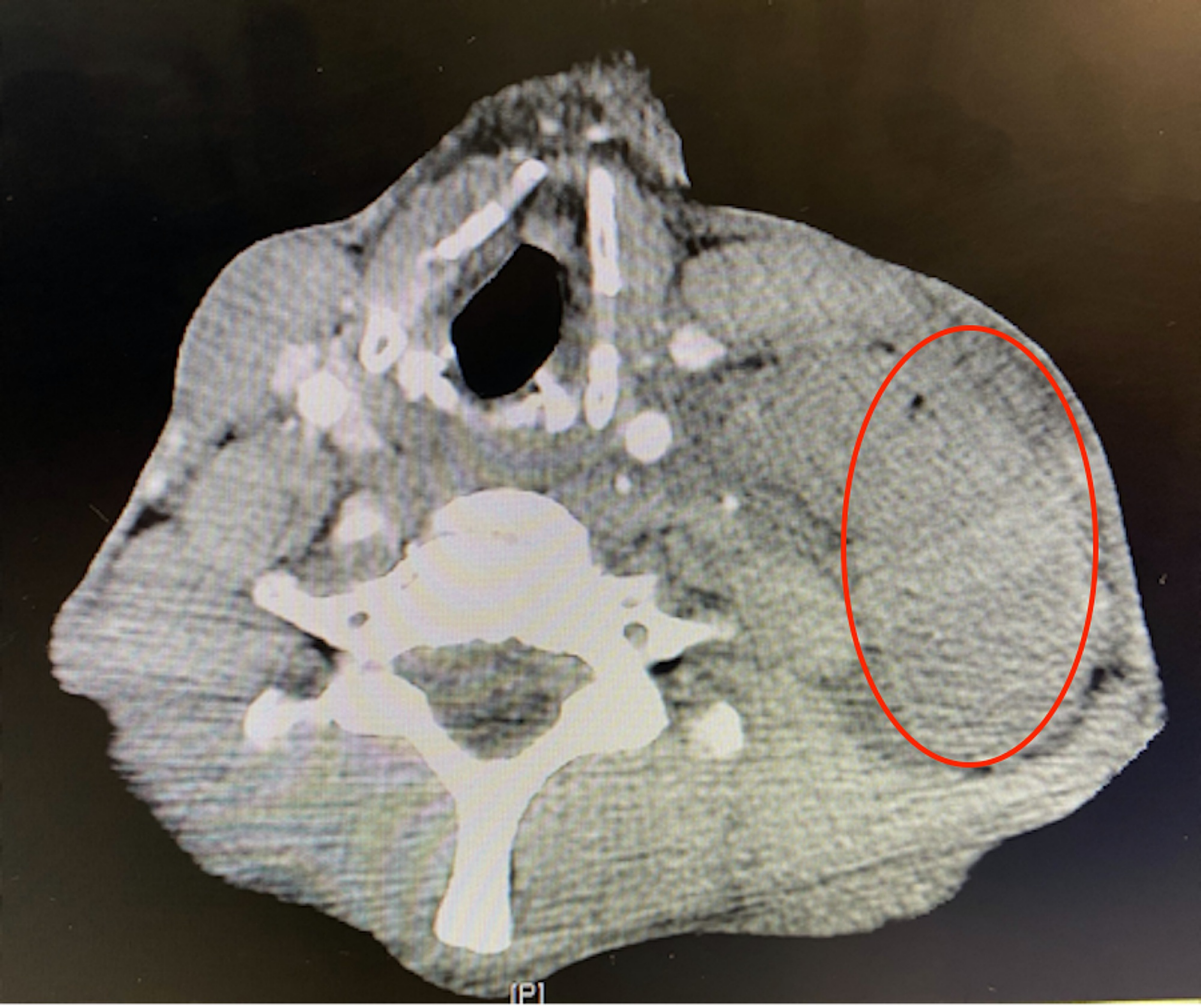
CT demonstrating left-sided neck solid mass (red circle) CT, Computed tomography.
